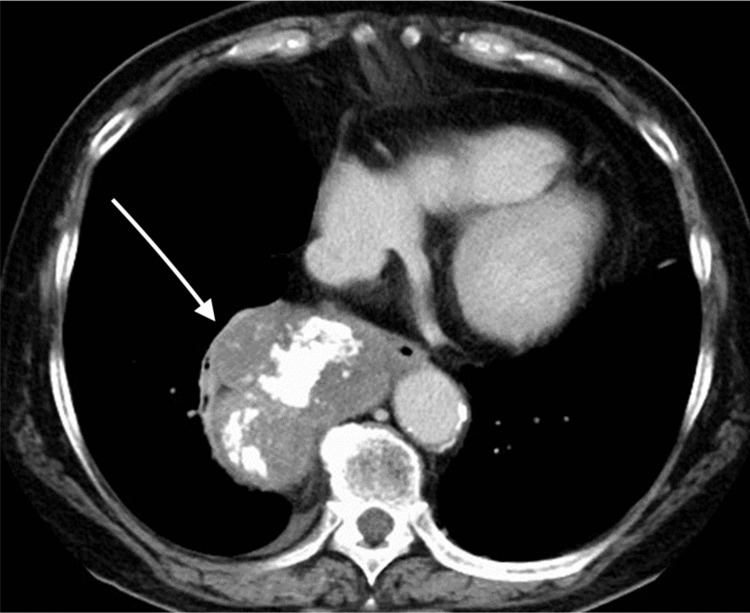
Chest computed tomography findings (mediastinal condition) A mass measuring 110 mm × 90 mm × 80 mm with internal calcification is observed on the right posterior mediastinum.
